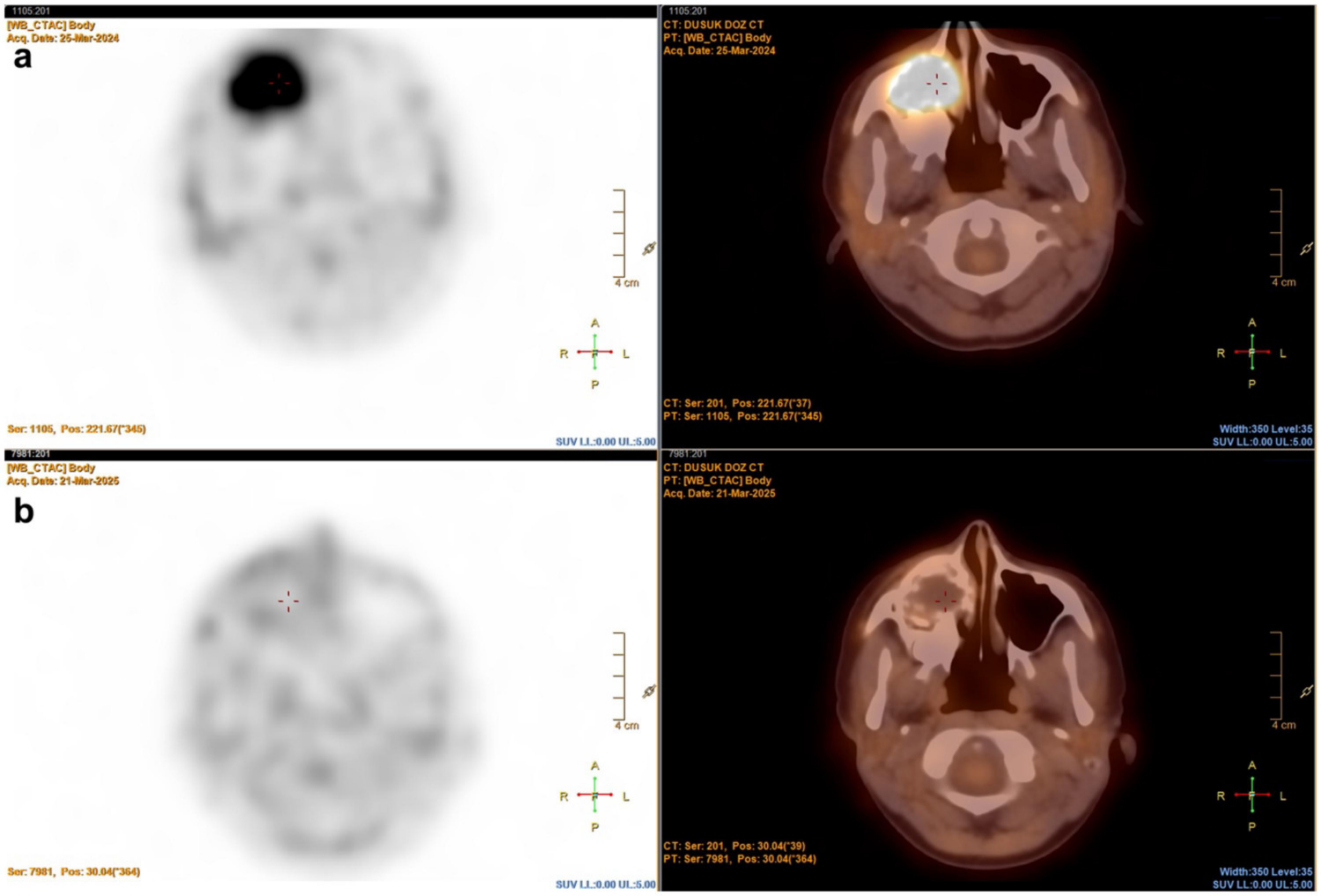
Comparative ^18^F-FDG PET/CT images before and after treatment. (a) Baseline ^18^F-FDG PET/CT scan (acquired on March 25, 2024) demonstrating a hypermetabolic lesion in the right maxillary sinus (SUVmax = 6.2), consistent with metabolically active high-grade sarcoma. (b) Post-treatment ^18^F-FDG PET/CT scan (acquired on March 21, 2025) showing complete metabolic response with no residual FDG uptake at the lesion site.
